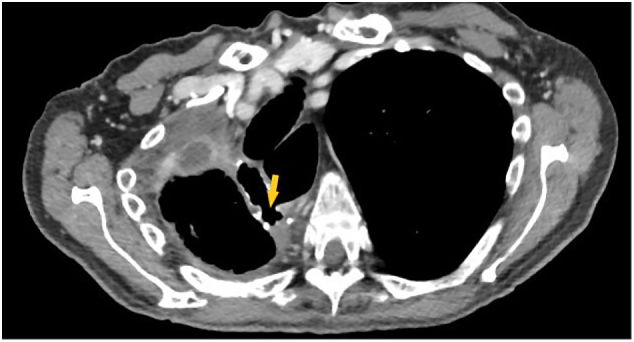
CT scan after the treatment with Eso-SPONGE®.
